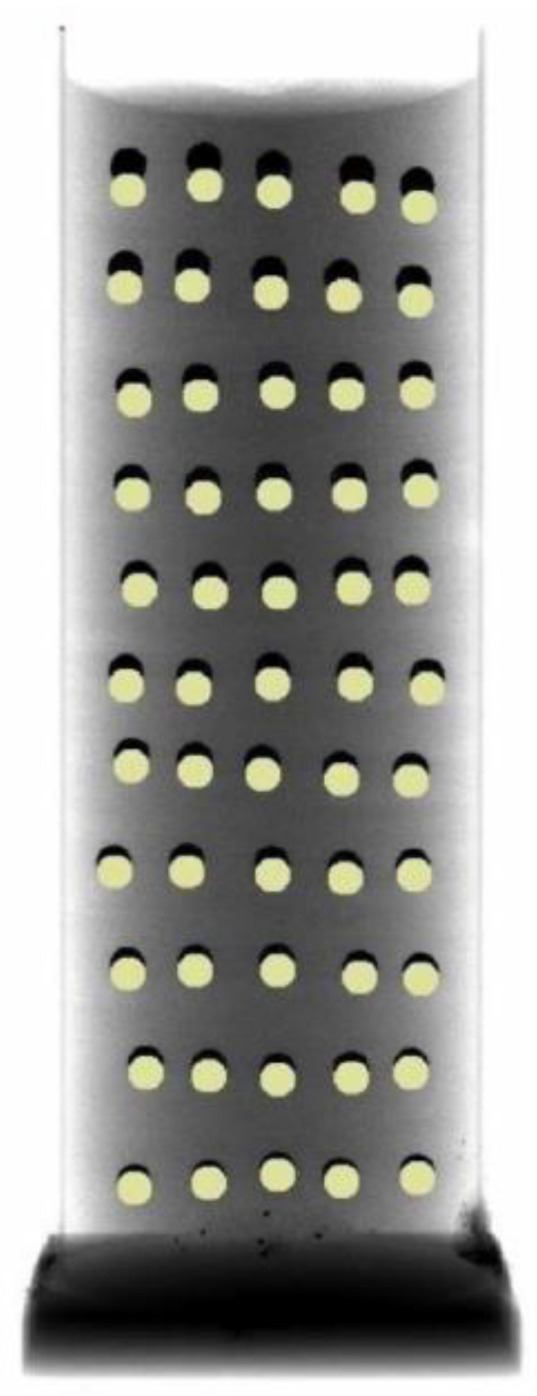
An X-ray tomography scan of the position of the balls before (black outline) and after (yellow outline) the compaction process.
